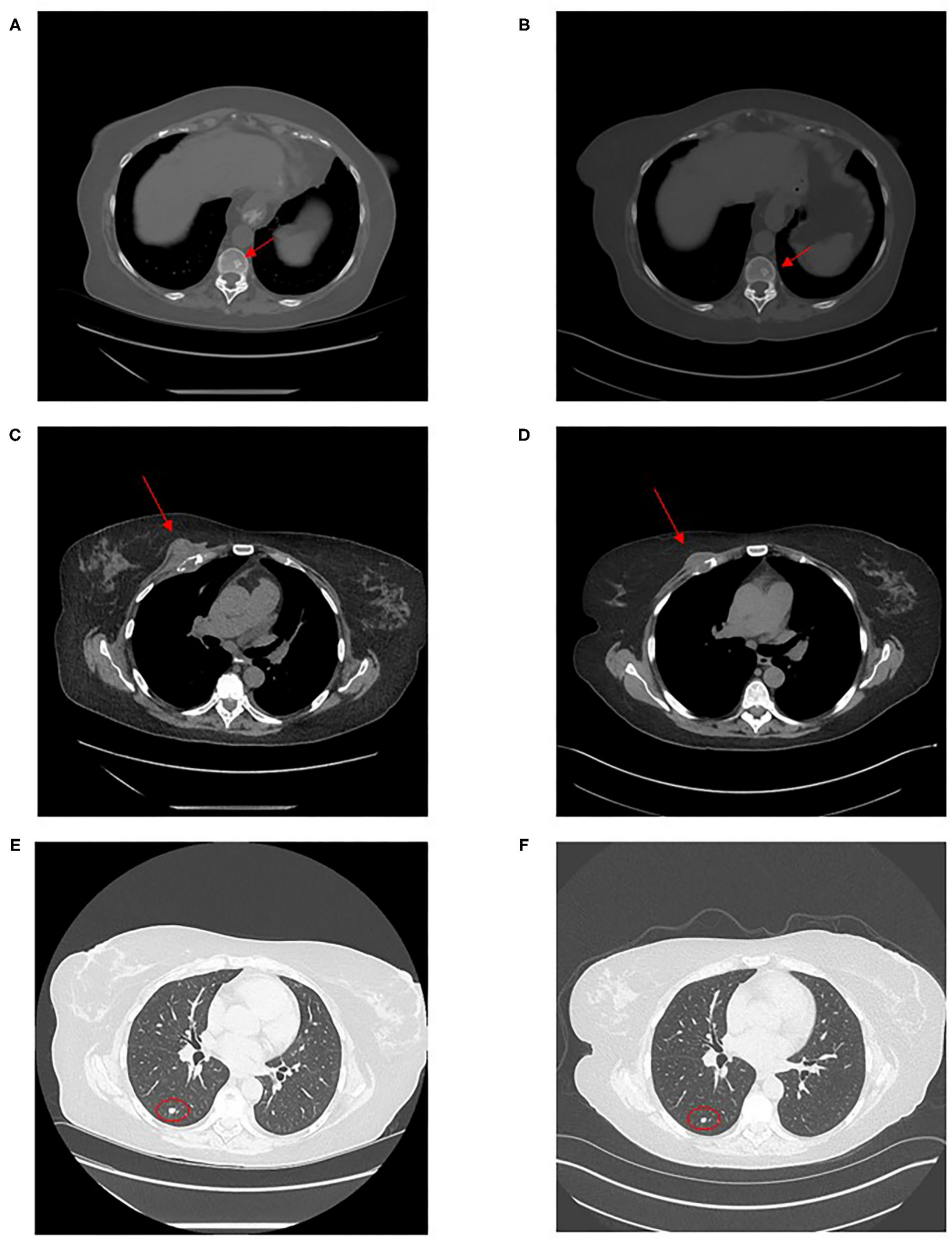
Computed tomography images of disseminated coccidioidomycosis lesions. Computed tomography images show: an axial sclerotic bone lesion in December 2020 (A) and after 4 months of fluconazole treatment in April 2021 (B), the chest wall mass in December 2020 (C) and in April 2021 (D) and a right lower lobe lung nodule in December 2020 (E) and in April 2021 (F).
